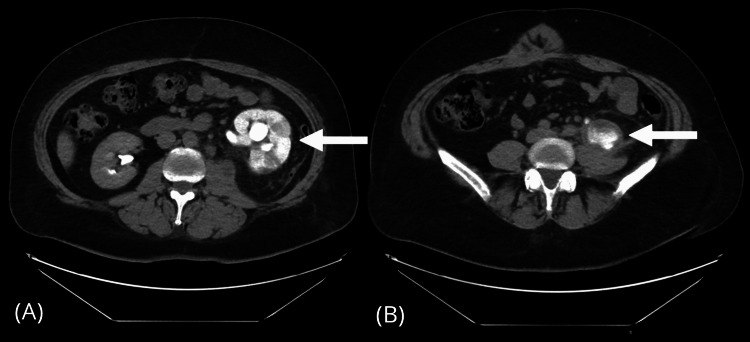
(A, B) CT scans of the patient during her last admission before surgery. (A) The left kidney showing retained contrast two hours post-administration. (B) Fluid collection on the patient’s left side indicating a urinoma with contrast. A linea alba hernia is also visible in the patient’s abdominal region.
